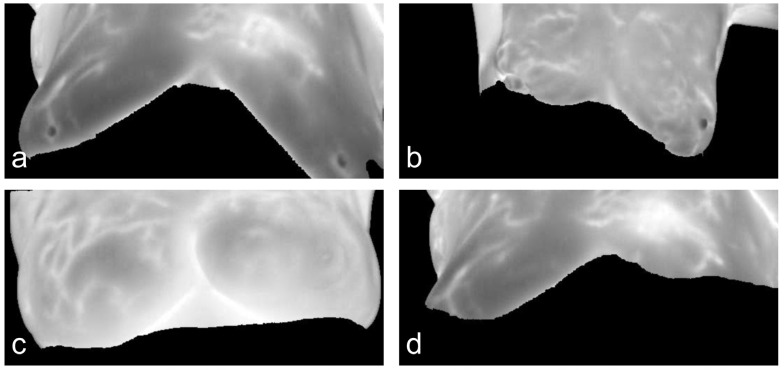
Representative MRI images of breast cancer patients. (a) A 48-year-old female patient with right breast cancer; (b) A 57-year-old female patient with bilateral breast cancer; (c) A 61-year-old female patient with right breast cancer; (d) A 55-year-old female patient with bilateral breast cancer.
